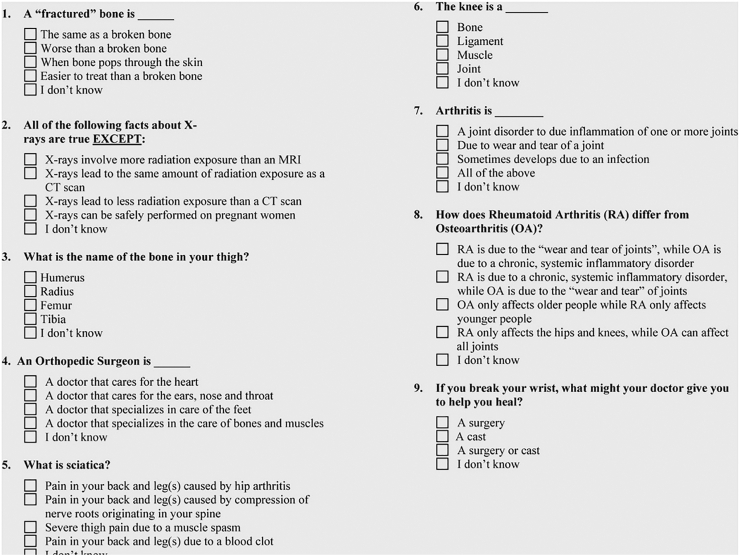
Survey questions included on the LiMP survey, a validated musculoskeletal health literacy scale. *LiMP*, Literacy in Musculoskeletal Problems; *MRI*, magnetic resonance imaging; *CT*, computed tomography.
